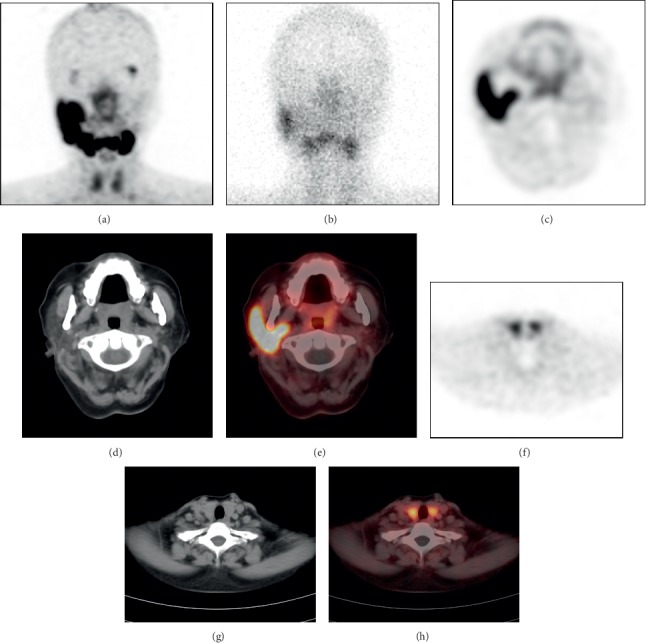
A 55-year-old woman (patient 1) with dry mouth and a dry right eye for half a year was initially diagnosed with sjogren's syndrome. ^68^Ga-PSMA-11 PET/CT MIP (a) and tomographic images (c–e) showed that the left parotid gland (SUVmax, 1.7) was smaller in volume and lower in density without ^68^Ga-PSMA-11 uptake than the right parotid gland (SUVmax, 9.2). SGS (b) confirmed that the uptake function of the left parotid gland was severely decreased (UR_left parotid_, 2.5; UR_right__parotid_, 10.0). In addition, it was found that the ^68^Ga-PSMA-11 uptake of the right lacrimal gland was lower than that of the left side (SUVmax_right__lacrimal gland_, 2.0; SUVmax_left__lacrimal gland_, 4.2). Combined with ^68^Ga-PSMA-11 PET/CT and the patient's medical history, the uptake function of the right eye was considered to be reduced. It was also found that the thyroid (f–h) density decreased with ^68^Ga-PSMA-11 diffuse uptake but without ^99m^TcO_4_^−^ uptake. The patient with a history of taking supplements had a 7-year history of Hashimoto's thyroiditis.
